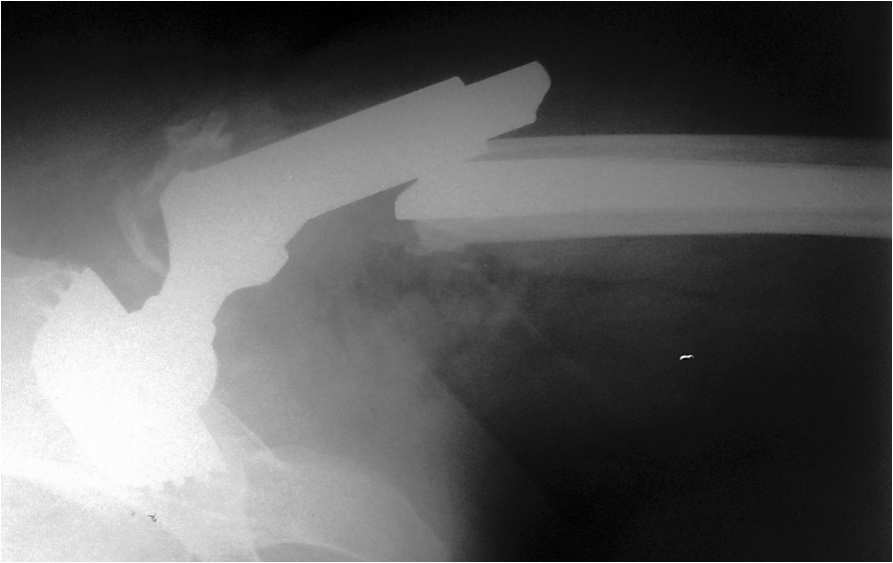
Plain radiograph of the fractured prosthesis.
